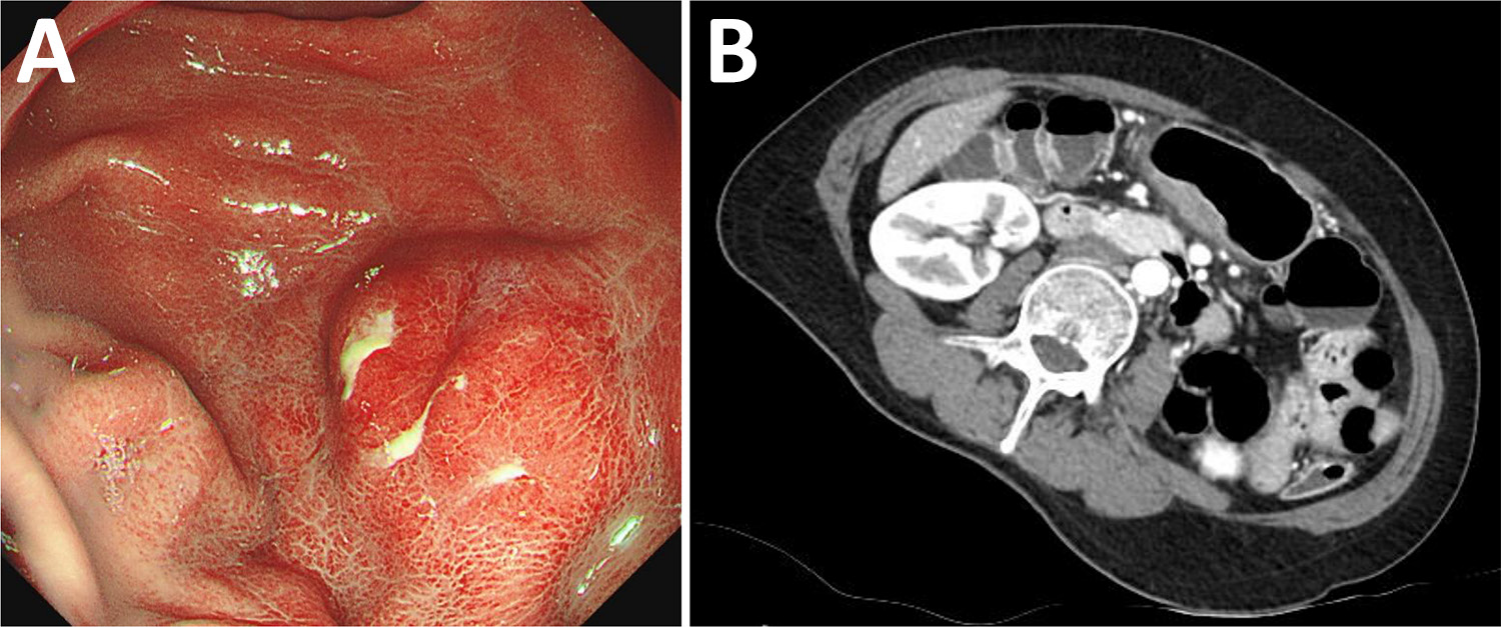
Gastric submucosal tumor in patient infected with *Dioctophyme renale* roundworm, South Korea, 2024. A) Gastroscopy showed a protruding mass-like lesion measuring 3 × 2 cm on the posterior gastric wall. B) Abdominal computed tomography showed enhanced wall thickening and a central low-attenuation area at the greater curvature of the gastric antrum.
